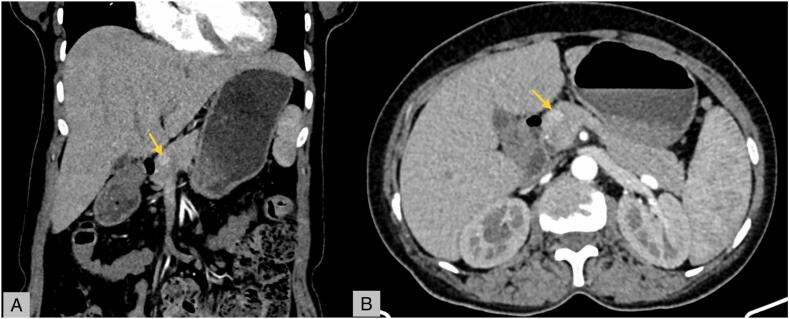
(A) CT Pancreatic protocol: Arterial phase – coronal view, (B) CT pancreatic protocol: Arterial phase – axial view.
